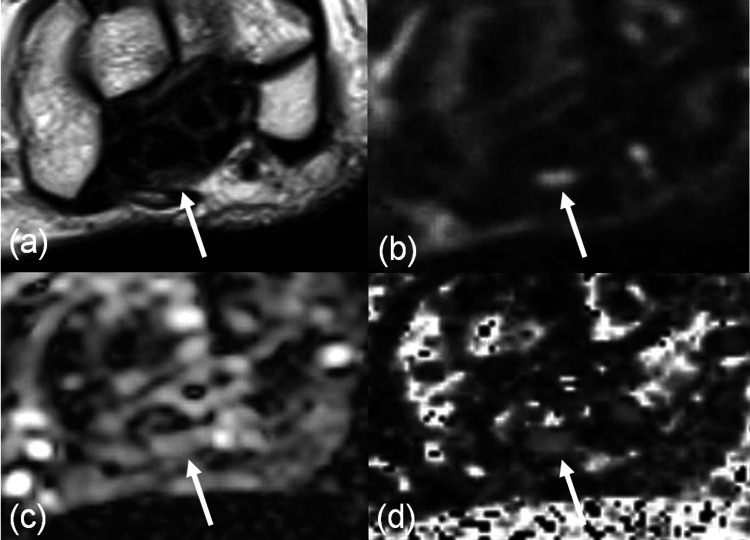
Diffusion tensor imaging of the wrist (NCV-abnormal case) FA: fractional anisotropy; ADC: apparent diffusion coefficient; NCV: nerve conduction velocity; T2WI: T2-weighted imaging; DWI: diffusion-weighted imaging A 69-year-old female patient presented with numbness in the right hand. NCV was abnormal, and the patient was ultimately diagnosed with carpal tunnel syndrome. Axial images of the right wrist at the level proximal to the pisiform bone are shown: (a) T2WI (4-mm thickness), (b) DWI (b = 1400, 4-mm thickness), (c) ADC map, and (d) FA map. The arrow indicates the median nerve. The ADC was 1.119 × 10⁻³ mm²/s, and the FA was 0.415
